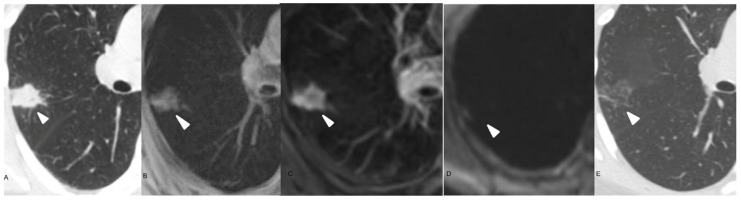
68-year-old male. (A) Axial non-enhanced CT chest, lung window, showing a consolidation in the right lung (arrow head). (B) Axial T1-weighted non-enhanced chest MRI exhibits a high signal lesion with poorly defined margins (arrow head). (C) Axial T2-weighted non-enhanced chest MRI. The lesion has predominantly high signal. (D) DWI sequence without remarkable restriction. (E) Three-month follow-up chest CT scan demonstrating significant lesion improvement.
